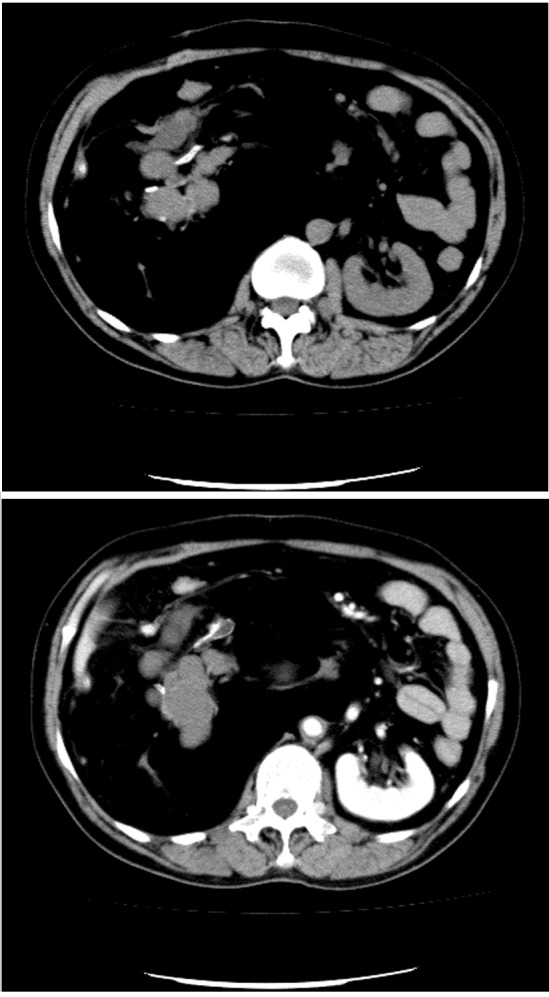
Computed tomography scans through the level of the celiac trunk reveals a giant retroperitoneal complex mass that was well-demarcated and contained a cystic room with fat, bone and tooth-like calcifications. The right kidney was encased by the tumor.
